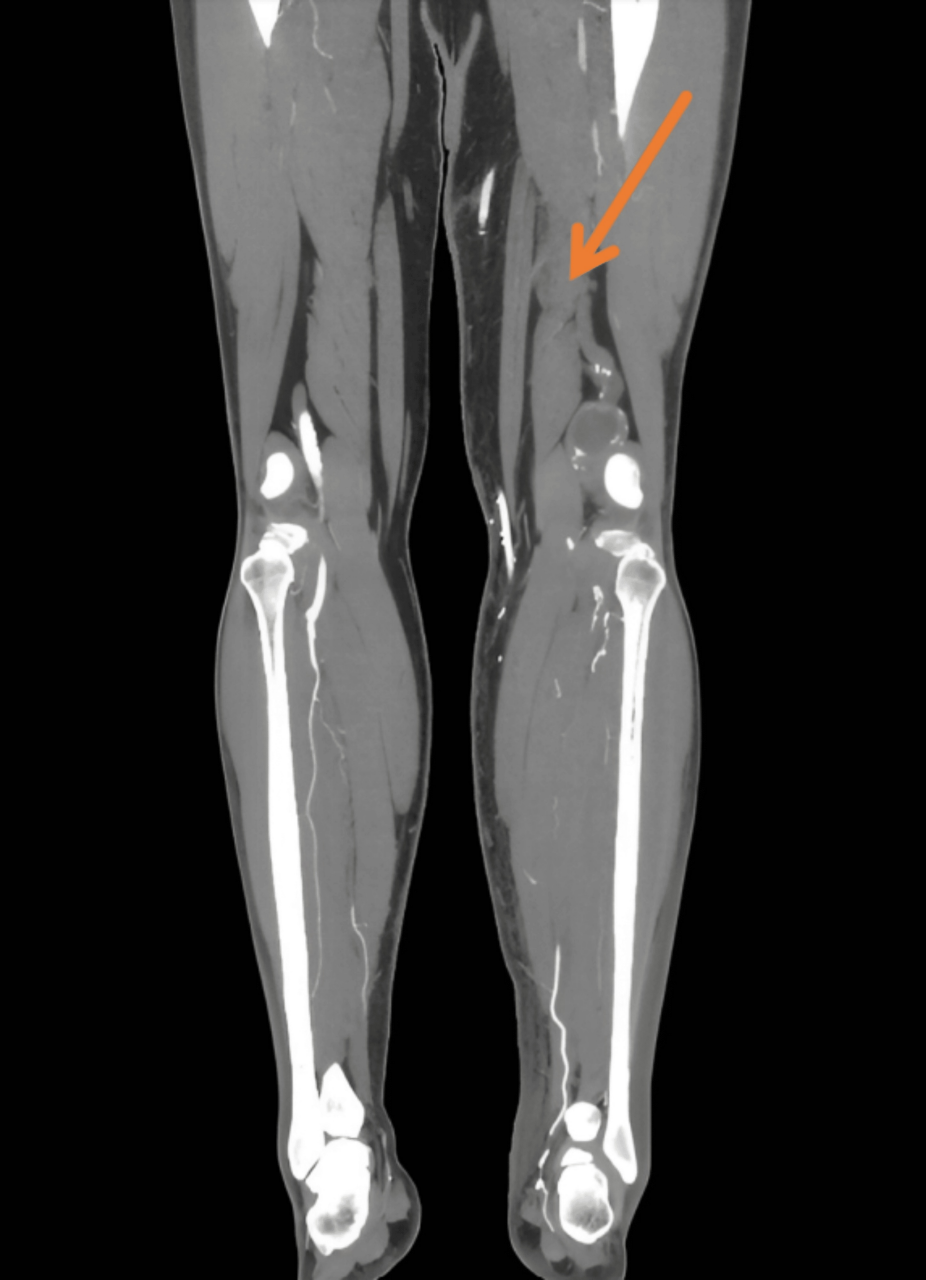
Computed tomography angiogram (coronal view) of the lower extremities showing some irregularity in the muscular contours of the sartorius and gracilis muscles (orange arrow).
